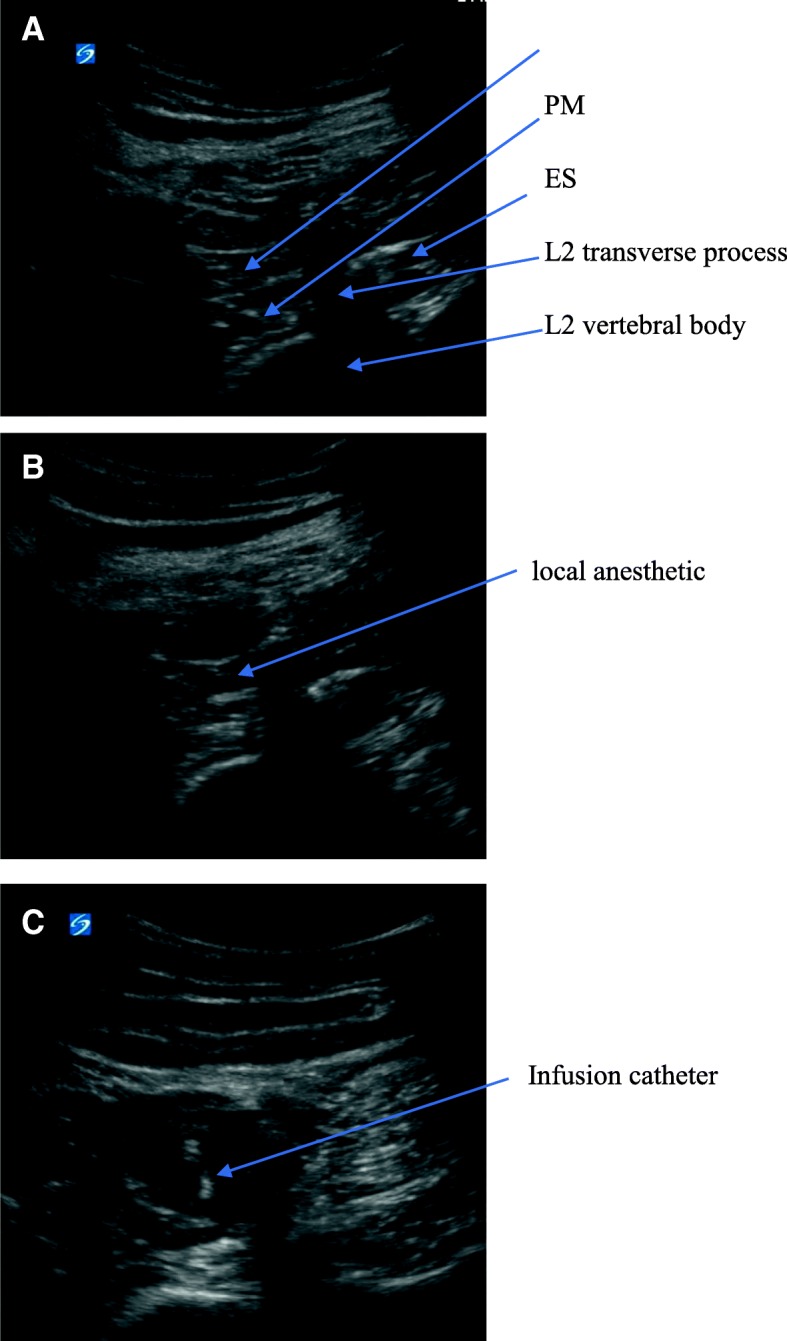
The ultrasound view of the CQLB. QL: Quadratus Lumborum PM: psoas major ES: erector spinae. a: ultrasound anatomical structure b: spread of local anesthetic. c: image of catheterization
